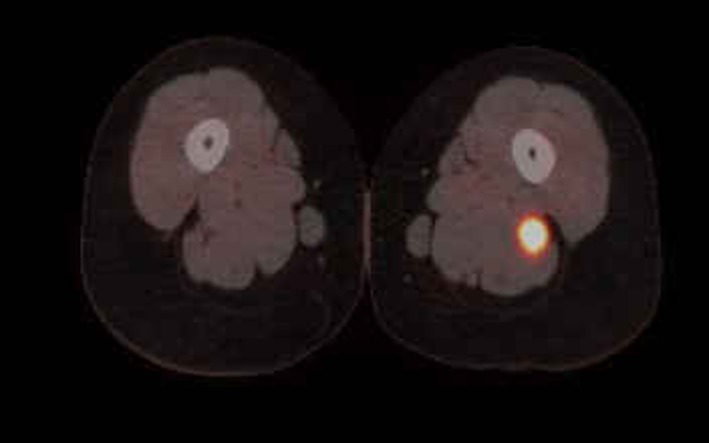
PET/CT clearly shows enlargement and increased uptake in the axial section.
